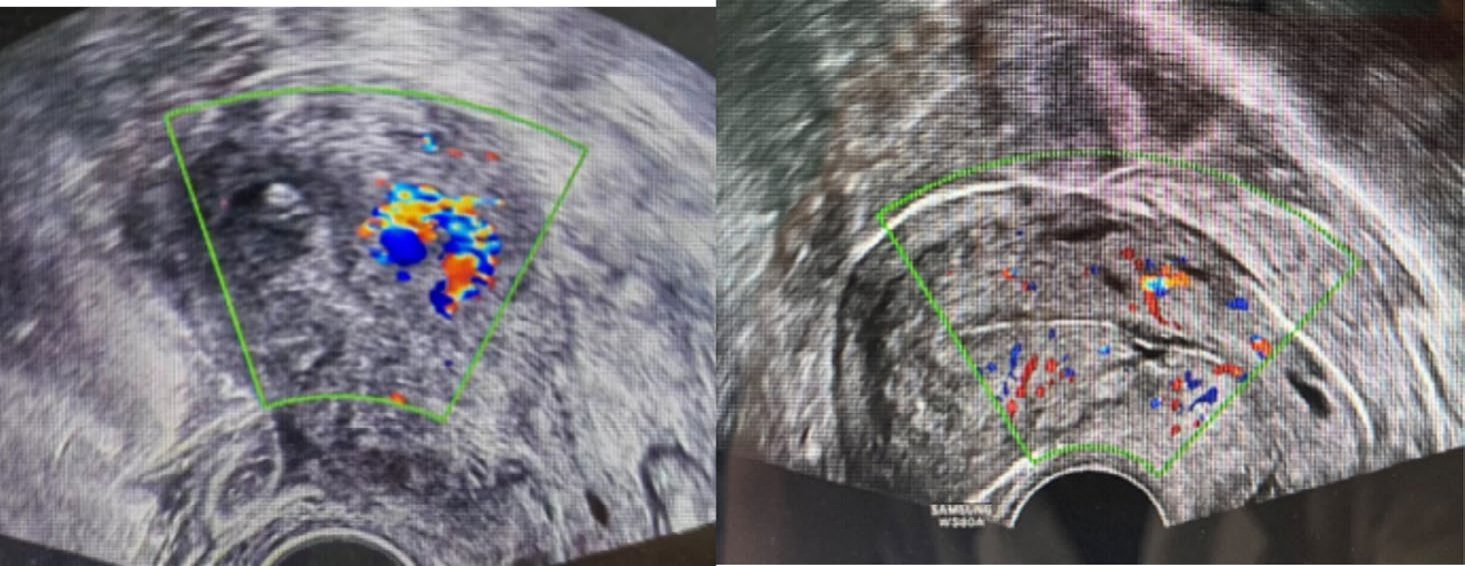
Changes in Doppler ultrasonography from pre‐diagnosis to post‐chemotherapy.
